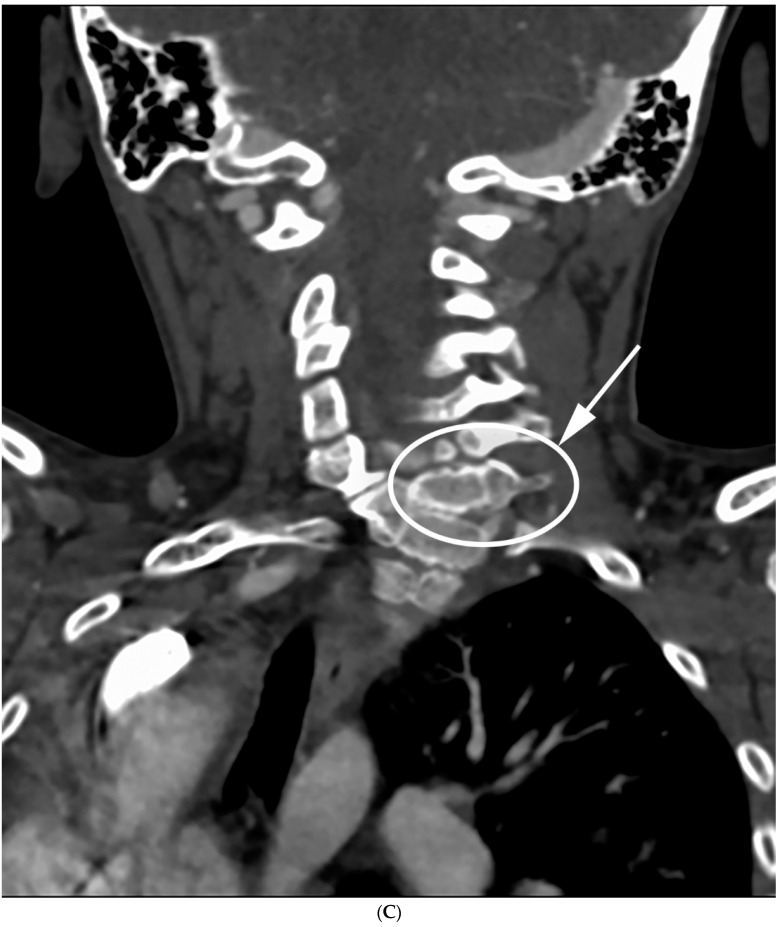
(A–C). An 8-year-old boy diagnosed with CCS with progression of cervical deformity and compensatory curve. (A) PA radiograph shows a C7 hemivertebra. (B) Lateral radiograph. (C) Coronal CT highlights the left C7 hemivertebra. Patients with thoracic or lumbar vertebral anomalies (such as hemivertebrae, butterfly vertebrae, etc.) were excluded from this study. This was to best ensure that any compensation in the thoracic or lumbar regions was not due to vertebral anomalies in those regions.
